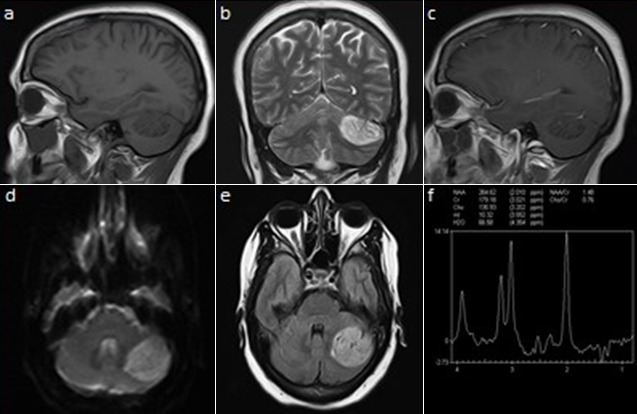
Head MRI: (A) sagittal T1-WI; (B) coronal T2-WI; (C) sagittal post contrast T1-WI; (D) diffusion WI; (E) FLAIR; (F) MRS: demonstrate left upper cerebellar hemisphere intra axial lesion, well defined, with striated appearance on T2-WI, which not enhance after gadolinium injection. This lesion appear with high intensity in FLAIR and DWI and moderately decreased choline (cho)/Cr ratio
